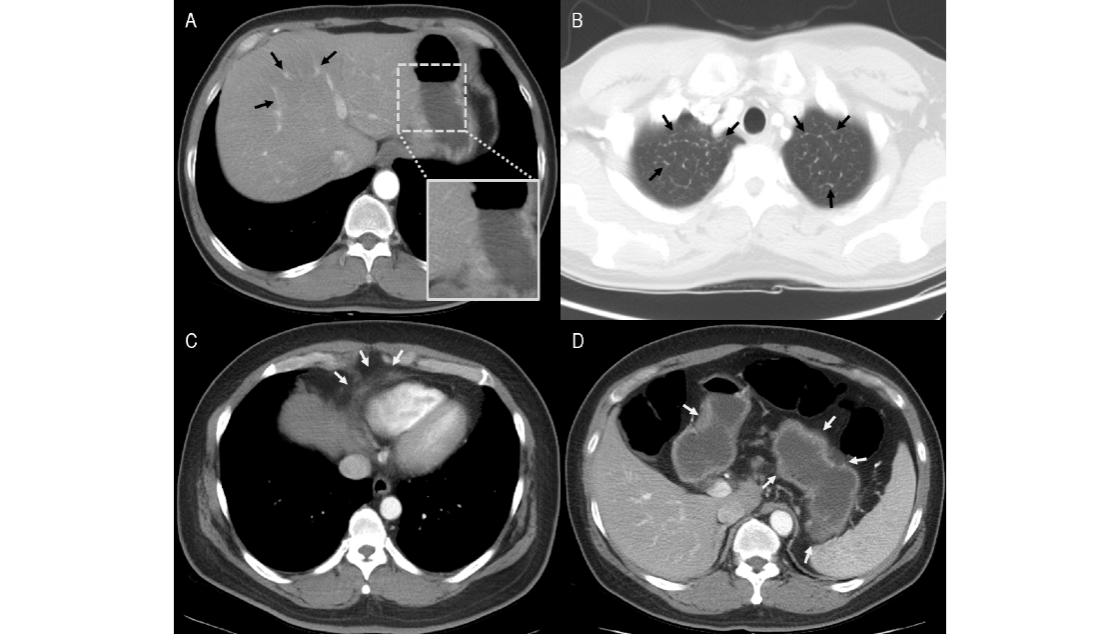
Obviously artificial body computed tomography images. A. Ill-defined margins and abnormal courses of intrahepatic vessels (arrows) in the liver. Note curvilinear structures (dotted rectangle) at the liver and stomach. B. Accentuated vascular markings in both upper lung apices (arrows). C. Abnormal infiltration in the pericardial fat (arrows). D. Irregular contours of the stomach body and antrum with blurred margins (arrows).
